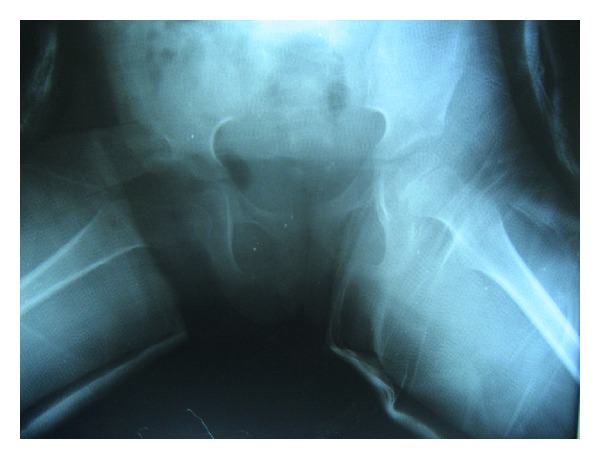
Plain radiograph after open reduction and Pemberton osteotomy.
